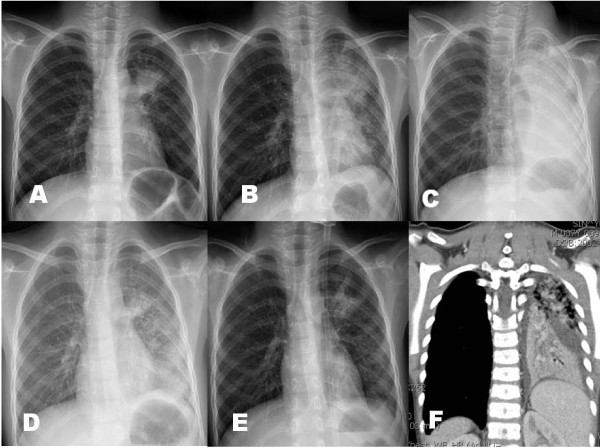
The chest radiographs of another patient who had rapidly progressive pneumonia during hospitalization (male, 7-year old); on admission (Figure 4-A), 2nd hospital day a.m. (Figure 4-B), 2nd hospital day p.m. (Figure 4-C), 3 h after MP treatment (Figure 4-D), 15 h after MP treatment (3rd hospital day, Figure 4-E), and a chest computed tomography finding on 2nd hospital day p.m. (Figure 4-F).
